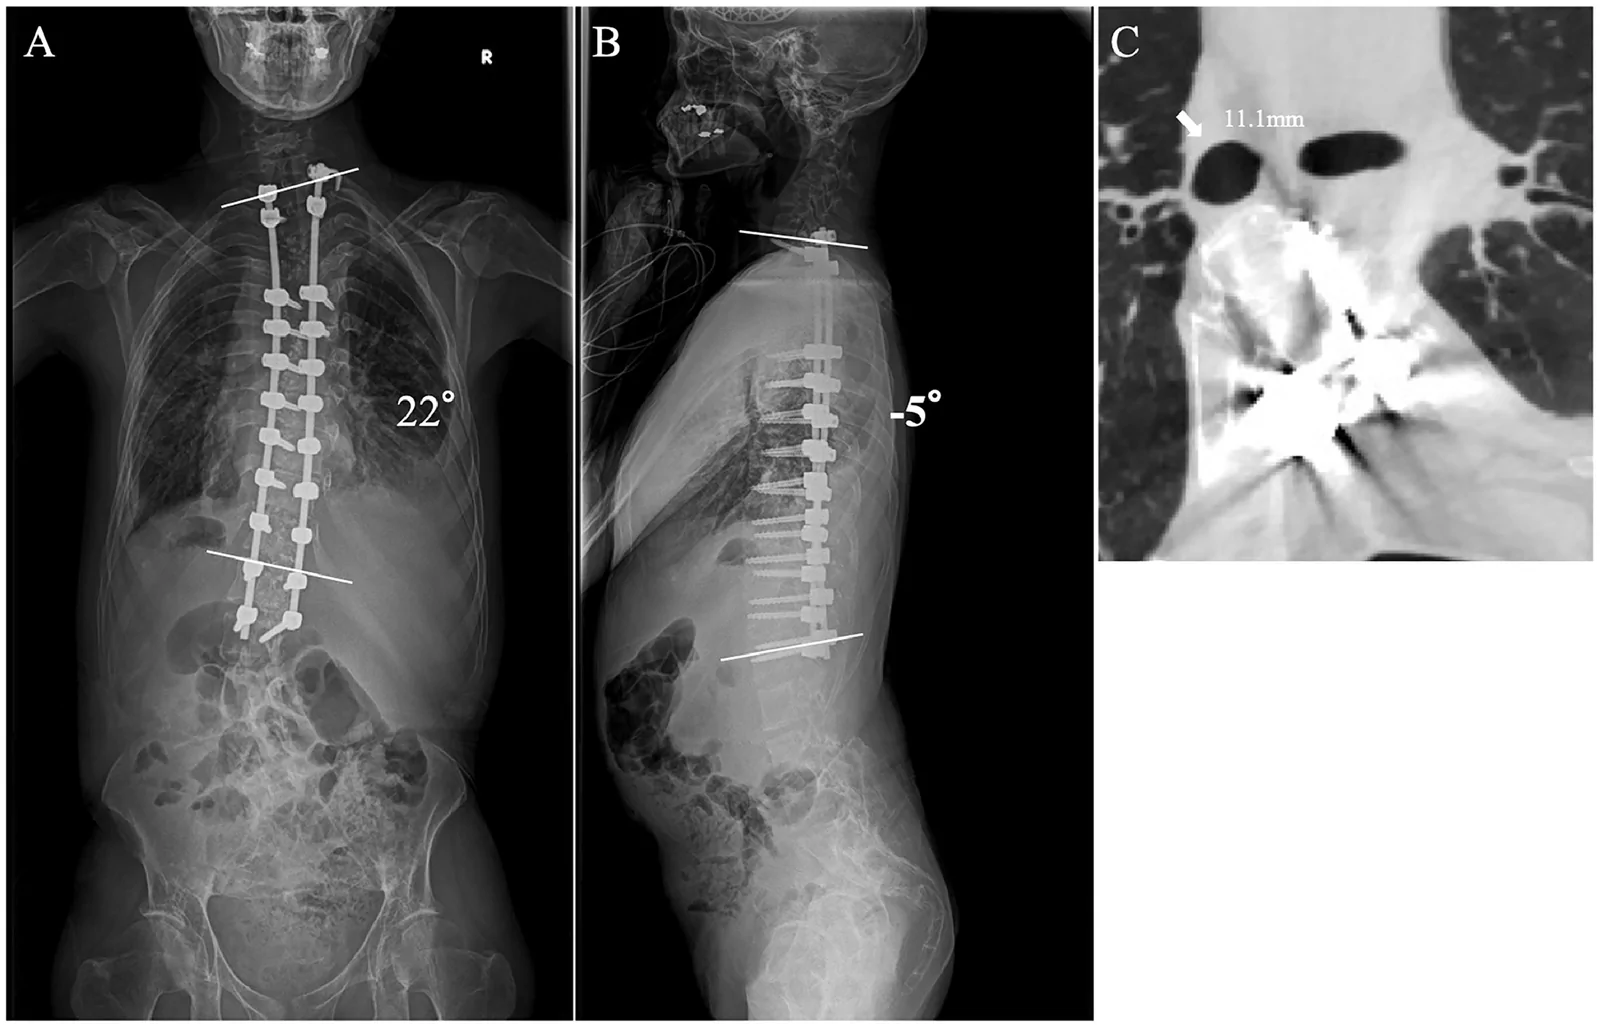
Postoperative upright whole-spine radiographs and upright MDCT at 4-year follow-up. (A) Postoperative upright anteroposterior radiograph. (B) Postoperative upright lateral radiograph. (C) Axial lung-window image of the right main bronchus in the upright position obtained from postoperative MDCT. Both the scoliosis and lordosis were corrected to 22 degrees from T1 to T12 (A) and −5 degrees from C7 to L1 (B). Postoperative upright MDCT showed increased lung volume of 3185 mL; a 77.5% increase compared to the preoperative level; and decompression of the right main bronchus (11.1 mm) (C).
